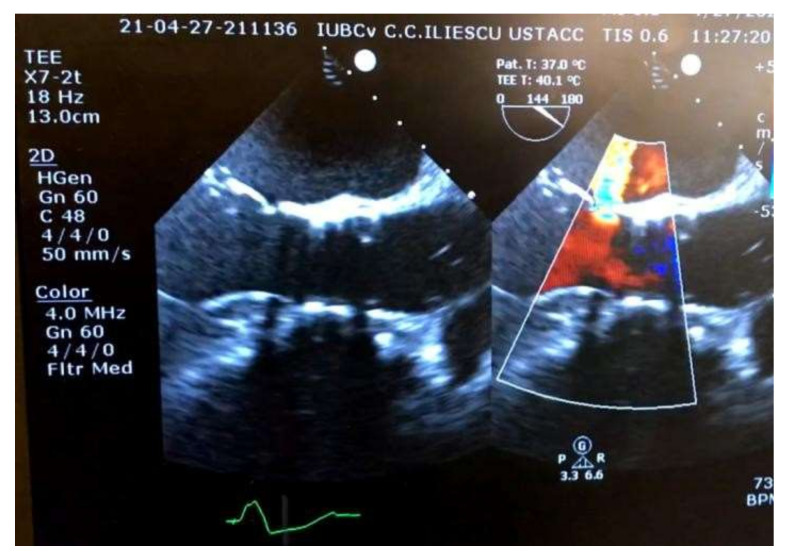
Intraprocedural transesophageal echocardiography—mitral regurgitation, AML perforation.
